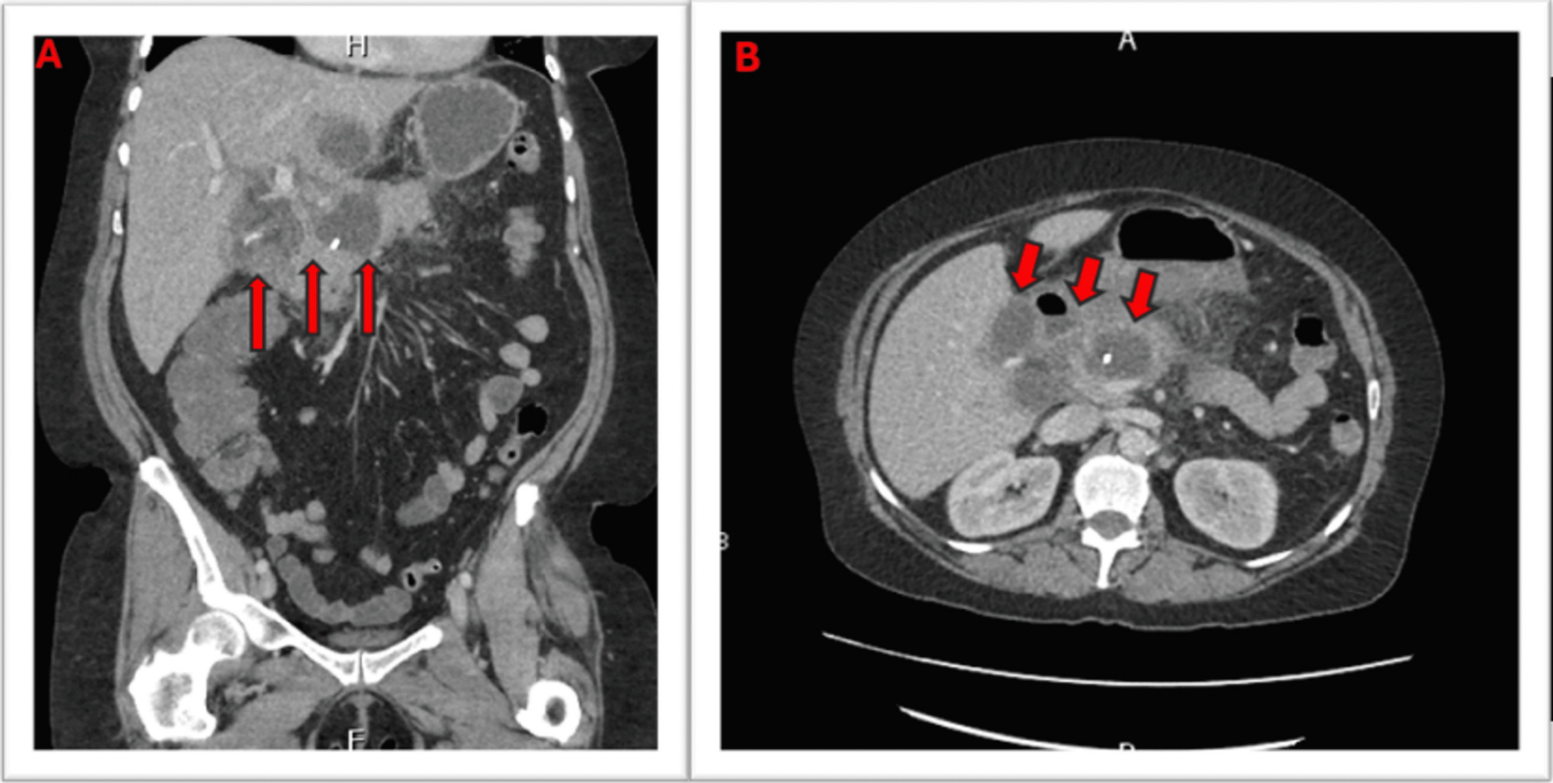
Contrast-enhanced CT abdomen and pelvis IV contrast-enhanced CT abdomen and pelvis: Interval placement of pancreatic stent. A slight decrease in the size of the fluid collection of the pancreatic neck. Upper abdominal/peripancreatic stranding and loculated fluid collection of the right upper quadrant are relatively stable. A small amount of intrapelvic free fluid has been resolved. Panel A depicts a coronal view, while panel B depicts an axial view.
